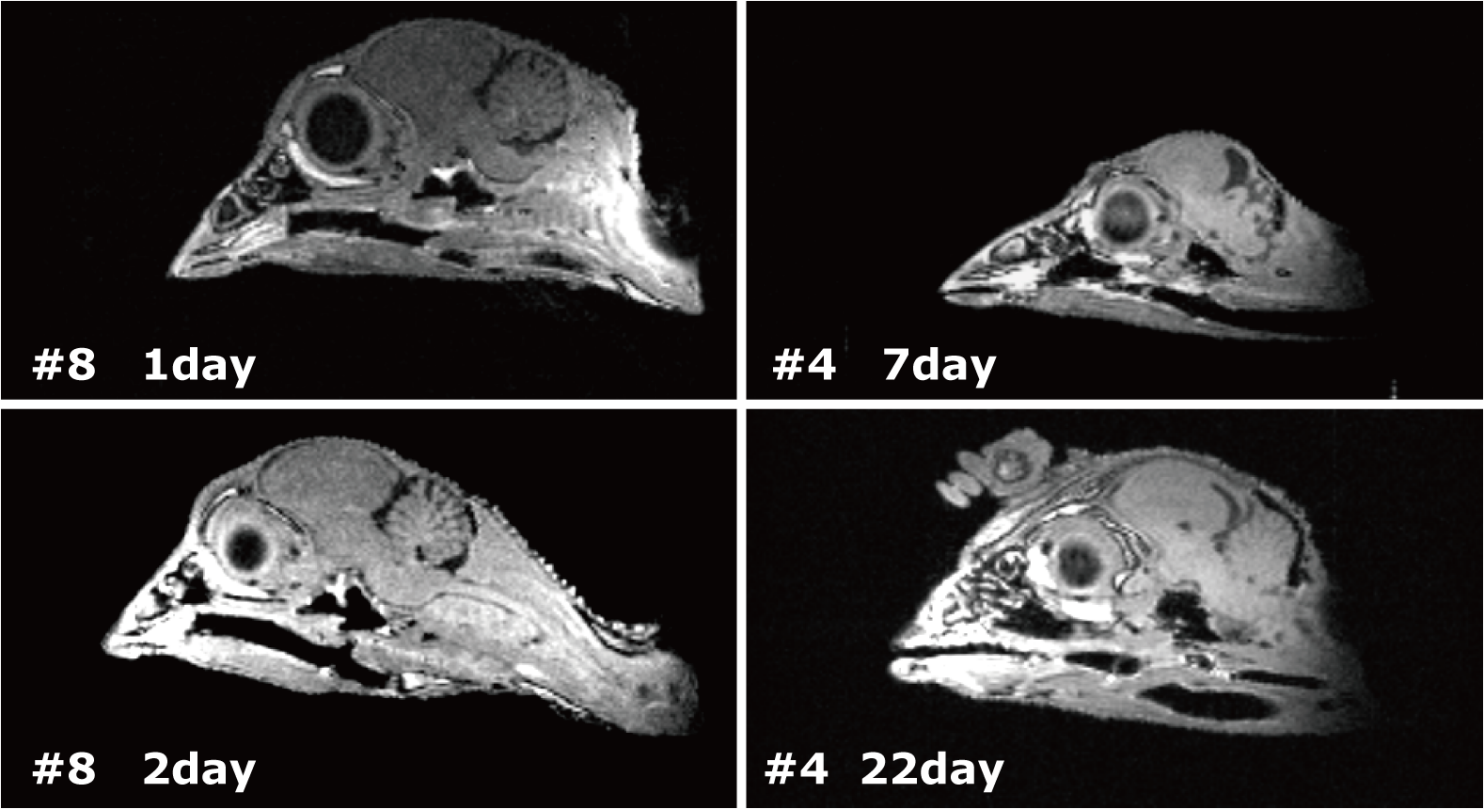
MRI images acquired by μMRI, MRmini SA (MRTechnology, Inc).
